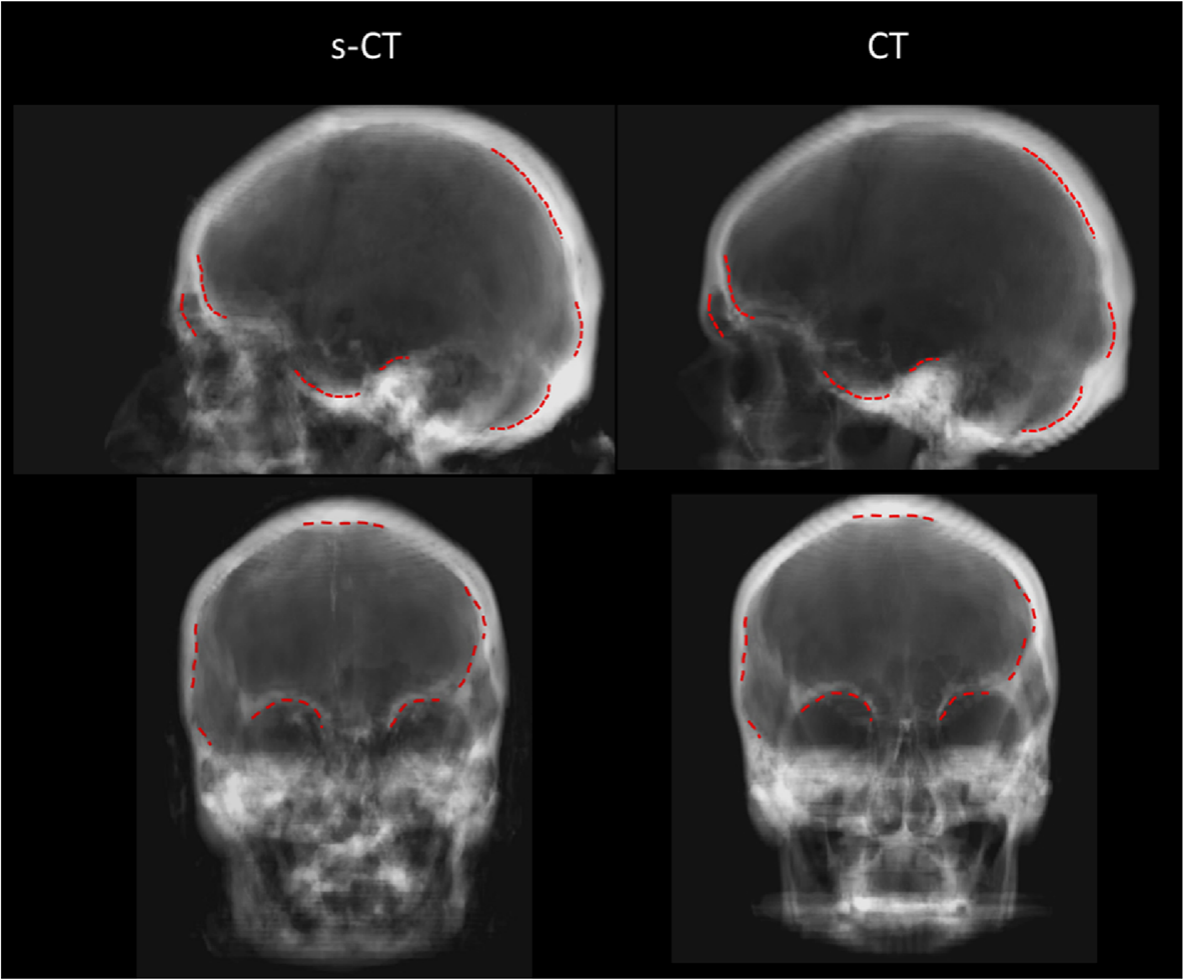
Lateral and anterior projections of the s-CT data (left) and CT data (right) for a representative patient. Structures deemed relevant for patient positioning are displayed using dashed red lines. The structures were delineated on the s-CT DRR and copied to the CT DRR. No obvious geometric differences between the two DRRs can be detected.
